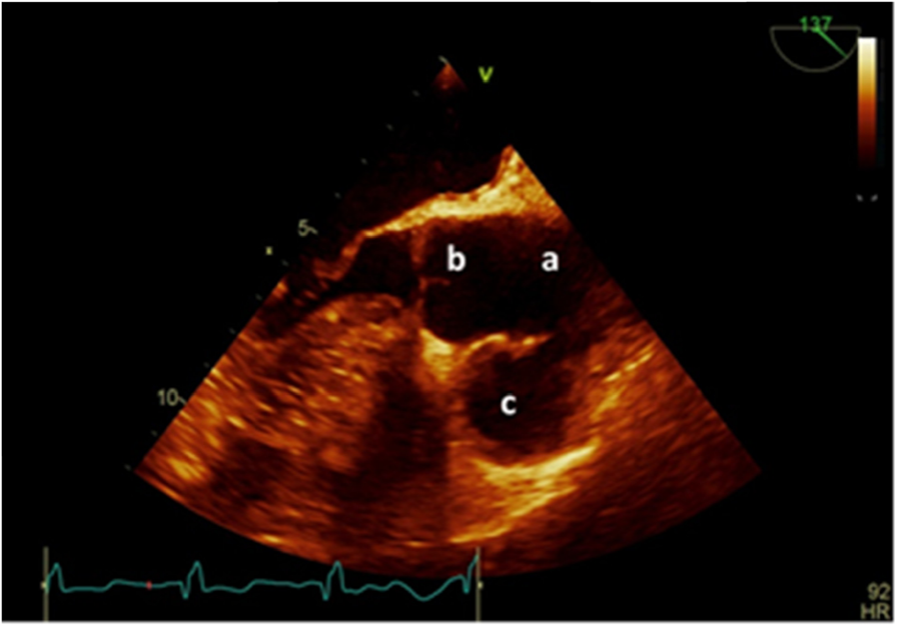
Trons esophageal echocardiography revealed Large pseudoaneurysm at right side of Aorta root (A) ascending, (B) sinus of Valsalva, (C) Pseudoaneurysm
